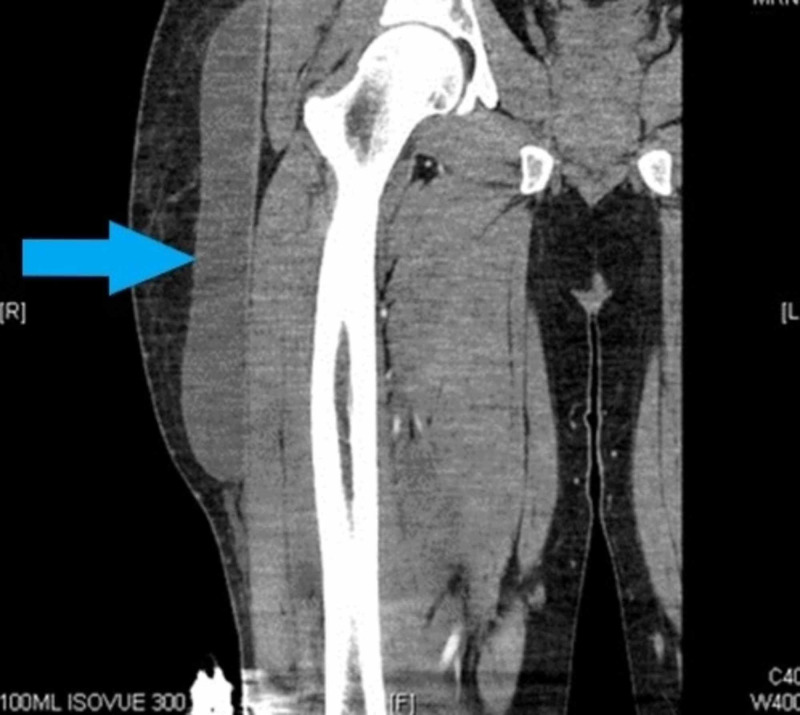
CT with IV contrast right lower extremity (coronal view) with a fluid collection within the deep aspect of the subcutaneous soft tissues
